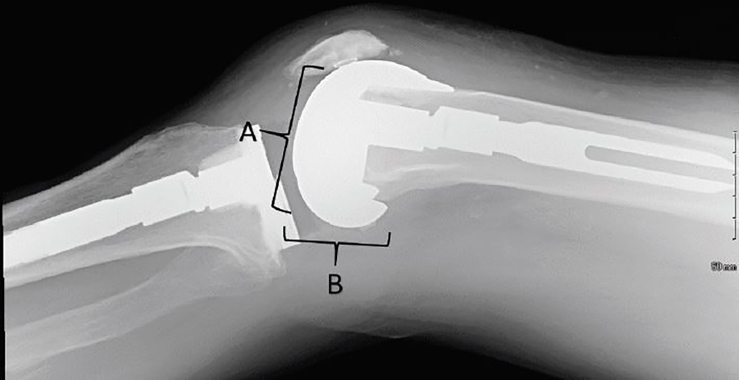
Post-reduction radiograph showing proper articulation of the prosthetic components. Prior to the reduction, the posterior aspect of the femoral component (B) articulated with the tibial component. After reduction, the inferior aspect of the femoral component (A) now articulates with the femoral component.
